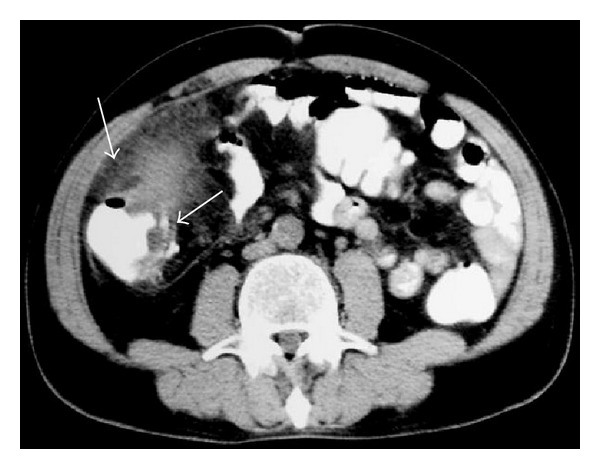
NHL. CT shows soft-tissue mass involving the ascending colon (arrows).
